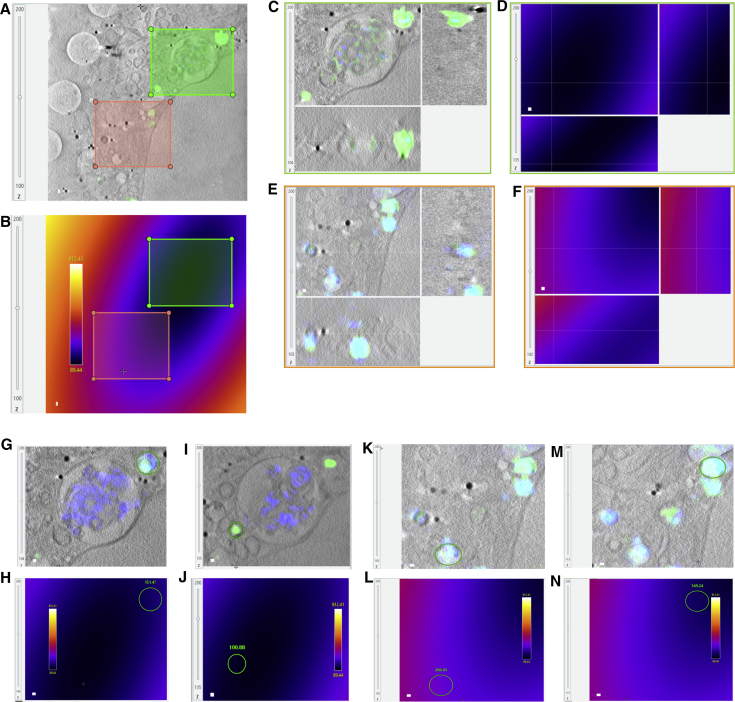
Estimation of error on reovirus datasets, related to Figures 6, S3, and S5 (A and B) In (A) is an XY slice of the 3D X-ray tomogram (extracted from 4 h post-infection datasets) and in (B) is the corresponding slice of the 3D error map generated using the point-set used for registration. The color codes for predicted average error is in nm, ranging from 89 to 813 nm in the full volume (two sub regions are selected for best and possible worst accuracy in this view (green shaded and red shaded respectively). Best accuracy areas overall lie near the gravity center of the point-set used for registration, dark blue). The scale bar is 100 nm. (C and D) In (C) are orthoviews of a crop of the merged dataset in the green sub region and in (D) is the corresponding crop in the error map. This area given the registration used, corresponds to the best accuracy we can obtain in this particular volume (89 to 150 nm). Scale bar is 100 nm. (E and F) In (E) is an orthoview of a crop of the merged dataset in the red sub region of (A), and in (F) is the corresponding crop in the error map. This orthoview is centered in an area with a predicted accuracy of 300 nm, which can be seen in the discrepancy in Z on the focused endosome. Scale bar is 100 nm. (G–N) Example of accuracy measured on finer areas within the error map for different endosomal arrangements. The circled areas indicate local 2D areas selected for which average predicted errors was measured, and their matching position on the merged dataset. The predicted error is displayed above these in the error map in nm. (G)–(J) and (K)–(N) are taken the green and red sub regions respectively. The scale bar is 100 nm.
